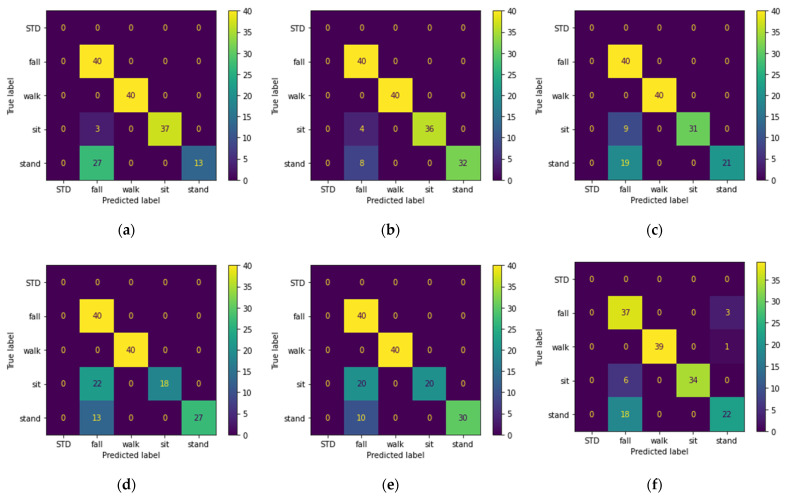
Confusion matrix of the activities in the unseen dataset predicted using: (a) MLP; (b) SVM; (c) Random Forest; (d) ERT; (e) KNN; (f) GTB; classifiers trained with top PCA components extracted from Doppler shift feature of the train dataset.
